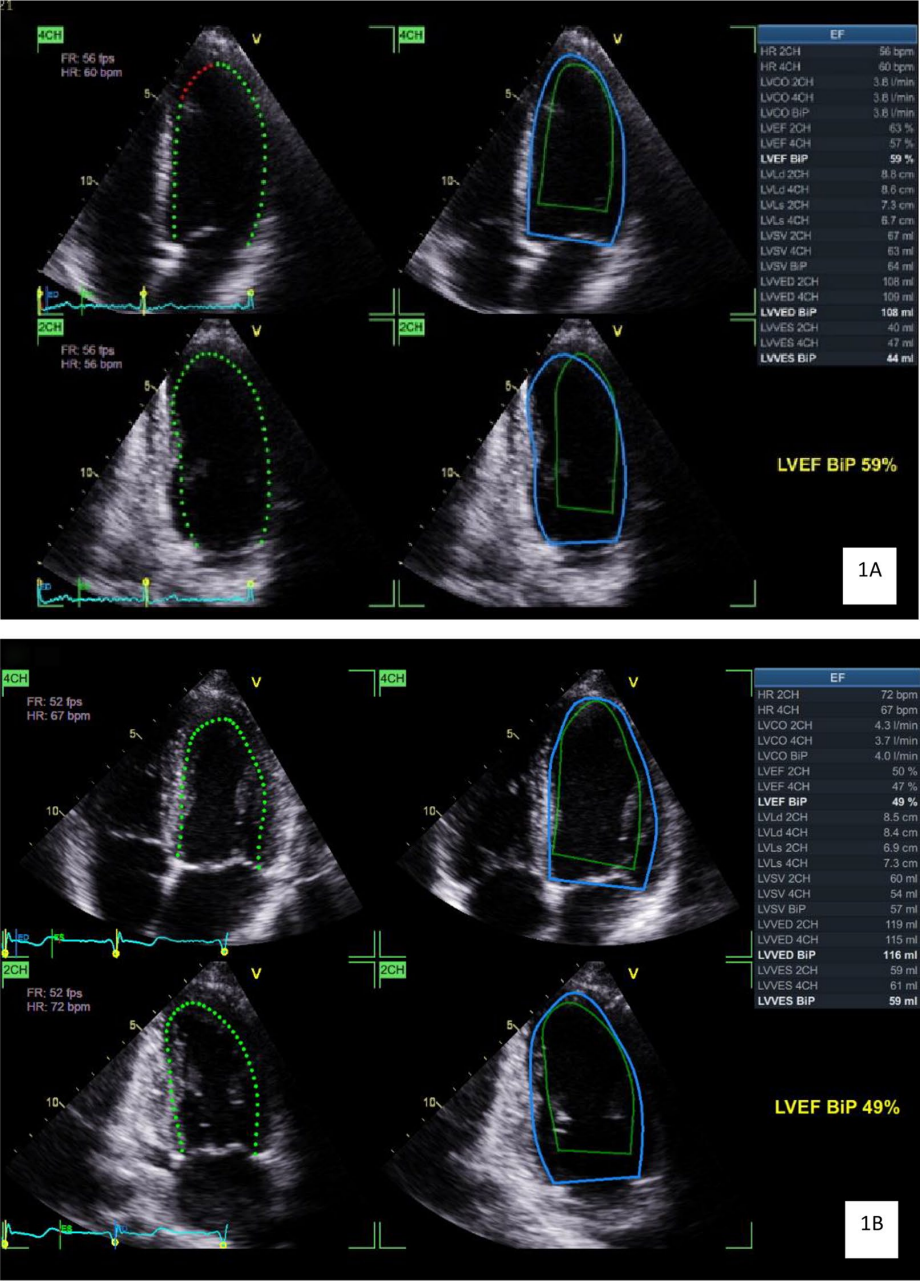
1A and 1B: Echocardiographic images demonstrating the measurement of left ventricular volume and ejection fraction from a general population participant (A) demonstrating a left ventricular end diastolic volume of 109mL and ejection fraction of 59% and in a jockey (B) demonstrating a larger left ventricular end diastolic volume of 116mL and lower ejection fraction of 49%. The dotted green line represents the instantaneous volume, the solid blue line represents end diastolic volume, and the solid green line represents end systolic volume. Abbreviations: 2CH – two chamber view, 4CH – four chamber view, BiP – biplane, bpm – beats per minute, cm – centimetres, CO- cardiac output, EF – ejection fraction, HR – heart rate, L – litres, LV – left ventricular, ml – millilitres, VED – volume at end diastole, VES – volume at end systole
